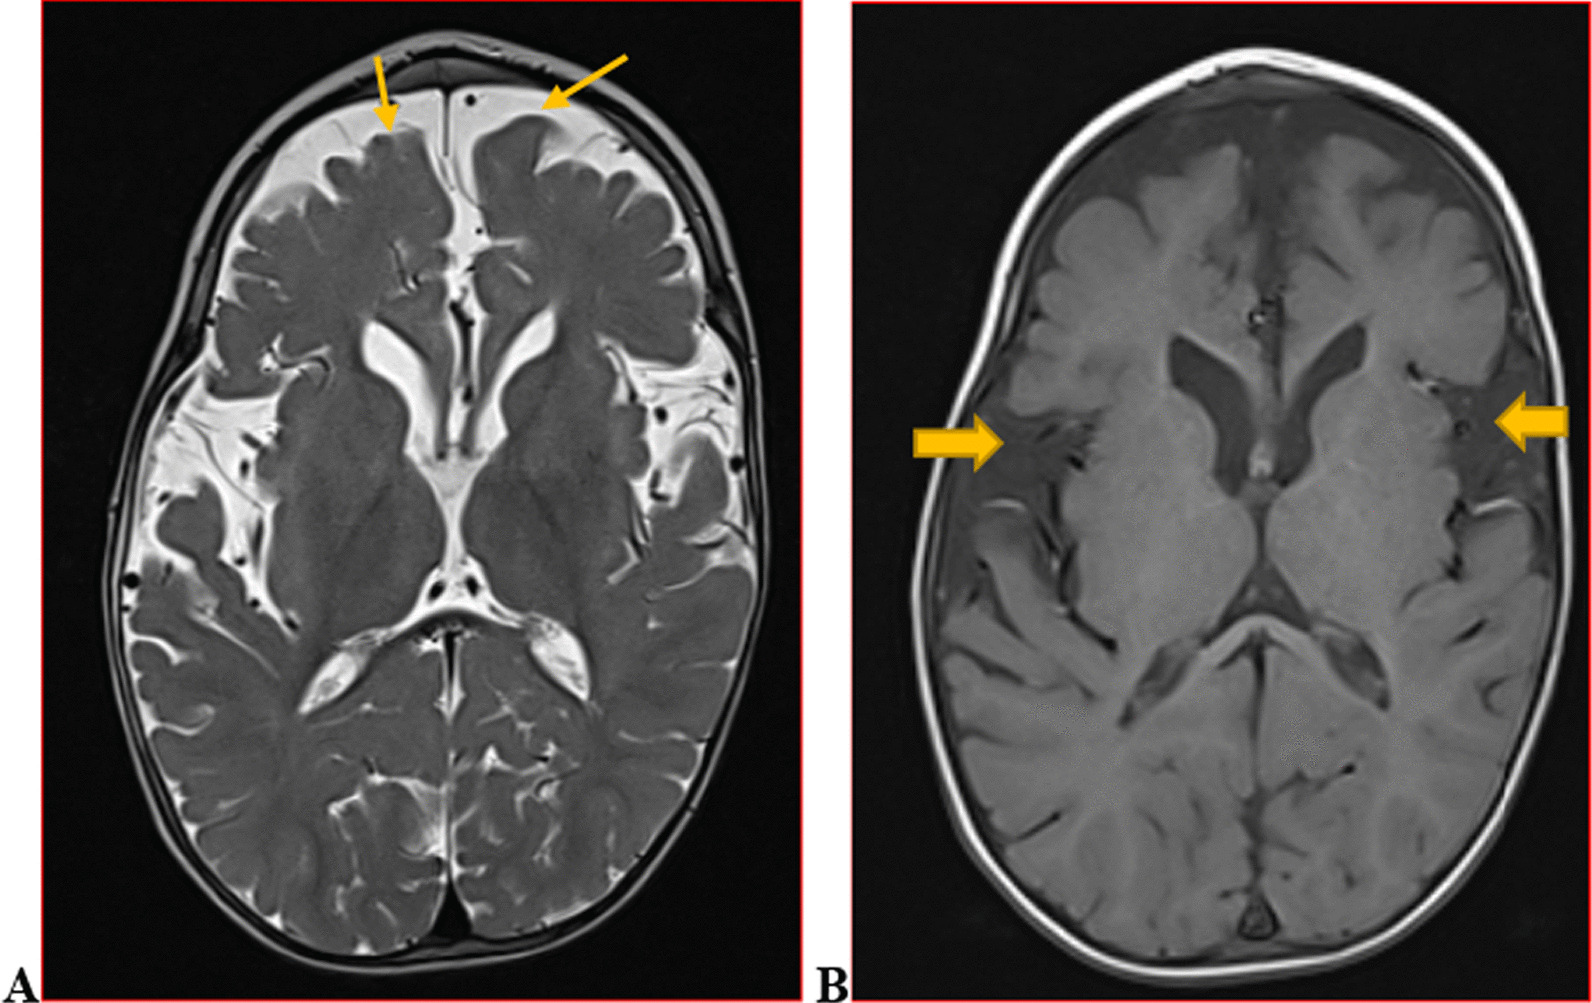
One and a half year old male with epilepsy and cranio-facial dysmorphism. Axial T2 (A) and T1 weighted (B) brain MRI showing delayed myelination with bilateral widened opercula and sylvian fissures with increased subarachnoid fluid (wide arrows).The frontal regions of the cerebral hemispheres are atrophied with enlarged anterior subarachnoid spaces(narrow arrows) in a case of type I glutaric aciduria
